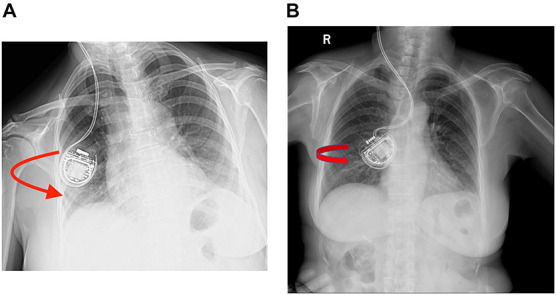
(A,B) Plain chest X-rays showing that the rechargeable implanted pulse generators (r-IPGs) were inside out. No fracturing or twisting of the leads was observed. The red arrow means that the r-IPG was inside out.
